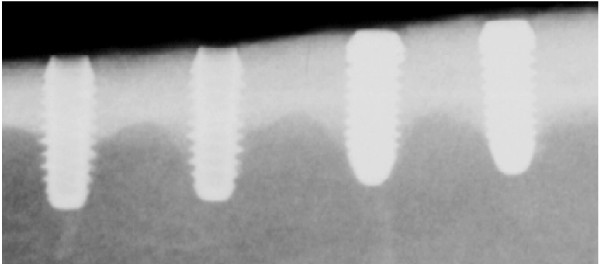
Radiograph showing titanium (left) and zirconia (right) implants inserted into the tibia of minipigs after 12 weeks of healing time.
